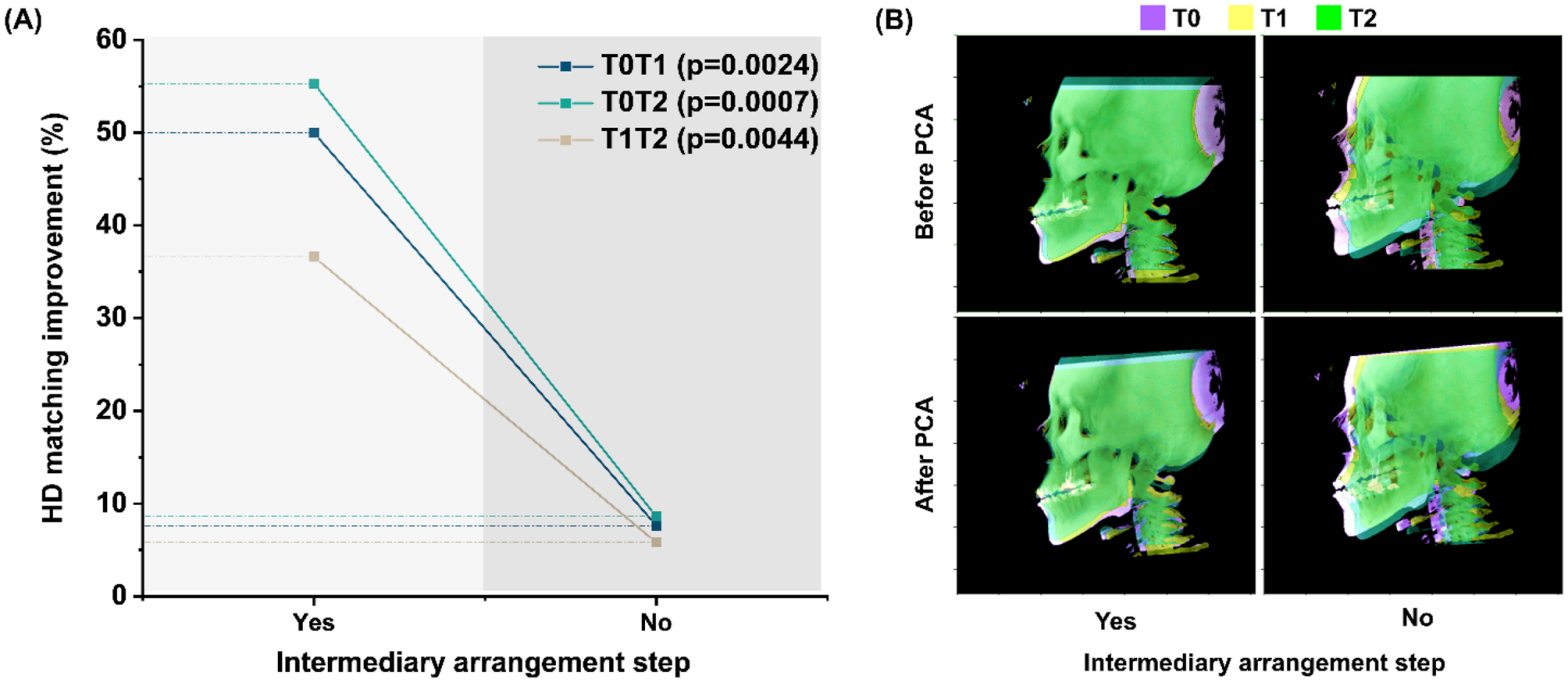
Comparison of the goodness of alignment of serial CBCT volumes with the intermediary arrangement step performed before the global axis is defined by measuring the (A) Hausdorff distance (HD) and (B) image overlay. PCA, principal component analysis.
